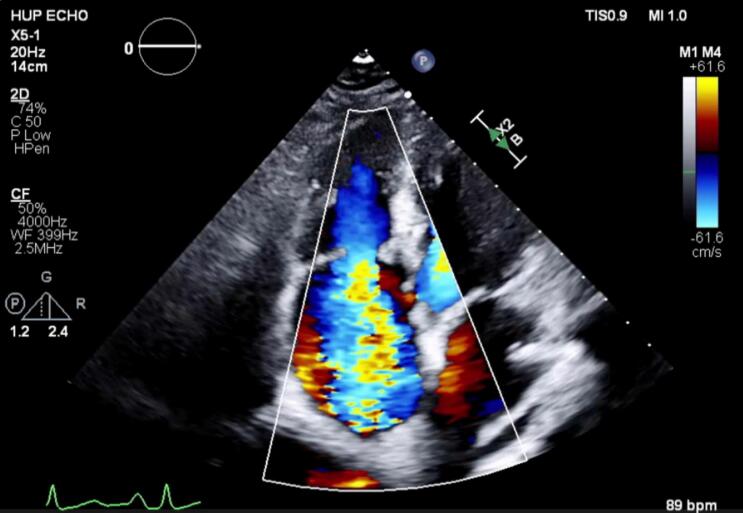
Transthoracic echocardiogram 4-chamber apical view with color Doppler flow demonstrating torrential tricuspid regurgitation.
